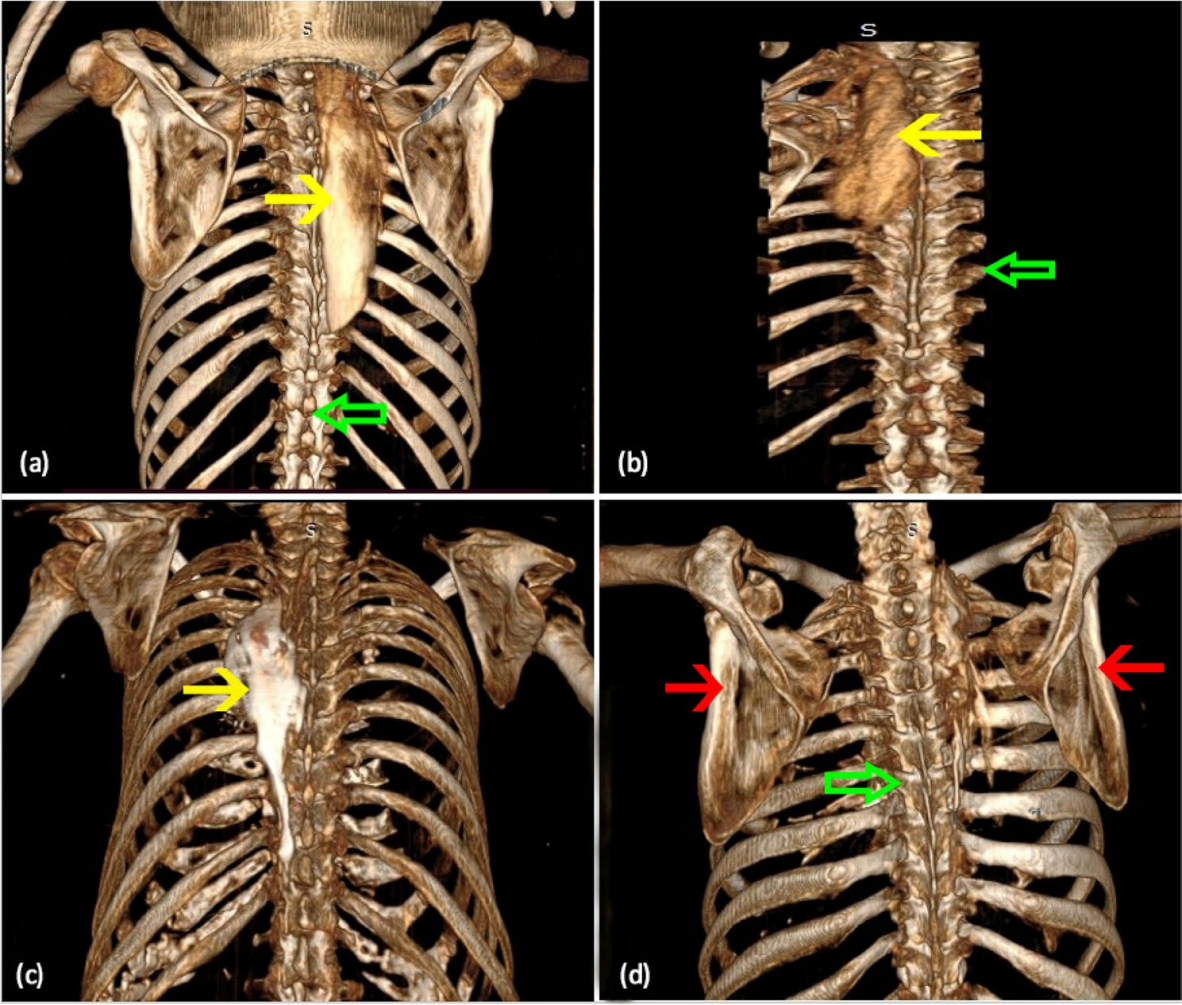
3D reconstruction of the computed tomography imaging of the thoracic region. Cuts (a-c) showing craniocaudal and lateral contrast spread (yellow arrows) in the thoracic region. Cut (d) shows the scapula (red arrows), and cuts (a, b, d) show the dorsal spine (green arrows)
